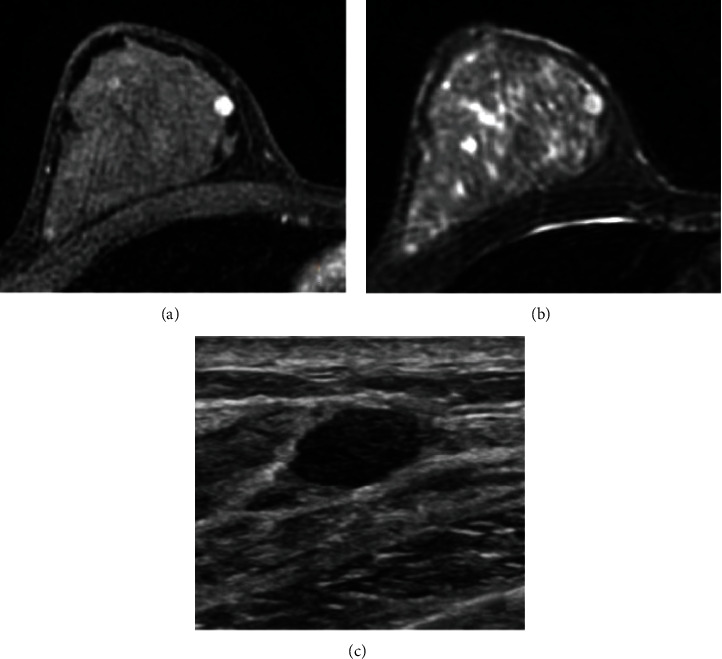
(a) MRI of the right breast with IV gadolinium shows an oval enhancing mass in the upper inner right breast middle depth; (b) T2-weighted images show bright signal, though less bright than cysts seen elsewhere in the breast. Slit-like spaces are not definitely appreciated, possibly due to the small size of the finding. (c) Second-look ultrasound shows an oval, microlobulated, hypoechoic mass in the upper inner right breast, corresponding with that seen on MRI.
